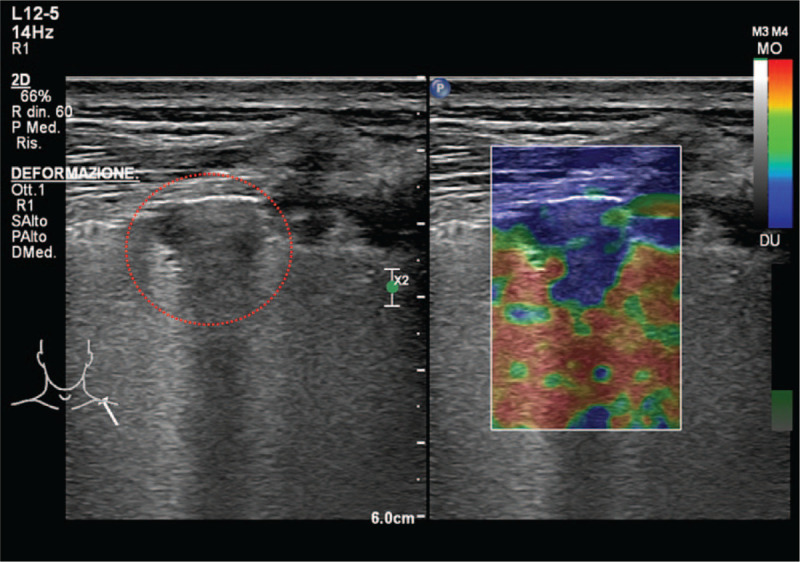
B-mode and strain elastosonography of sub-pleural lung consolidation; the lesion displayed a blue pattern >75% (hard lesion). The lung lesion is highlighted by the red dotted line.
